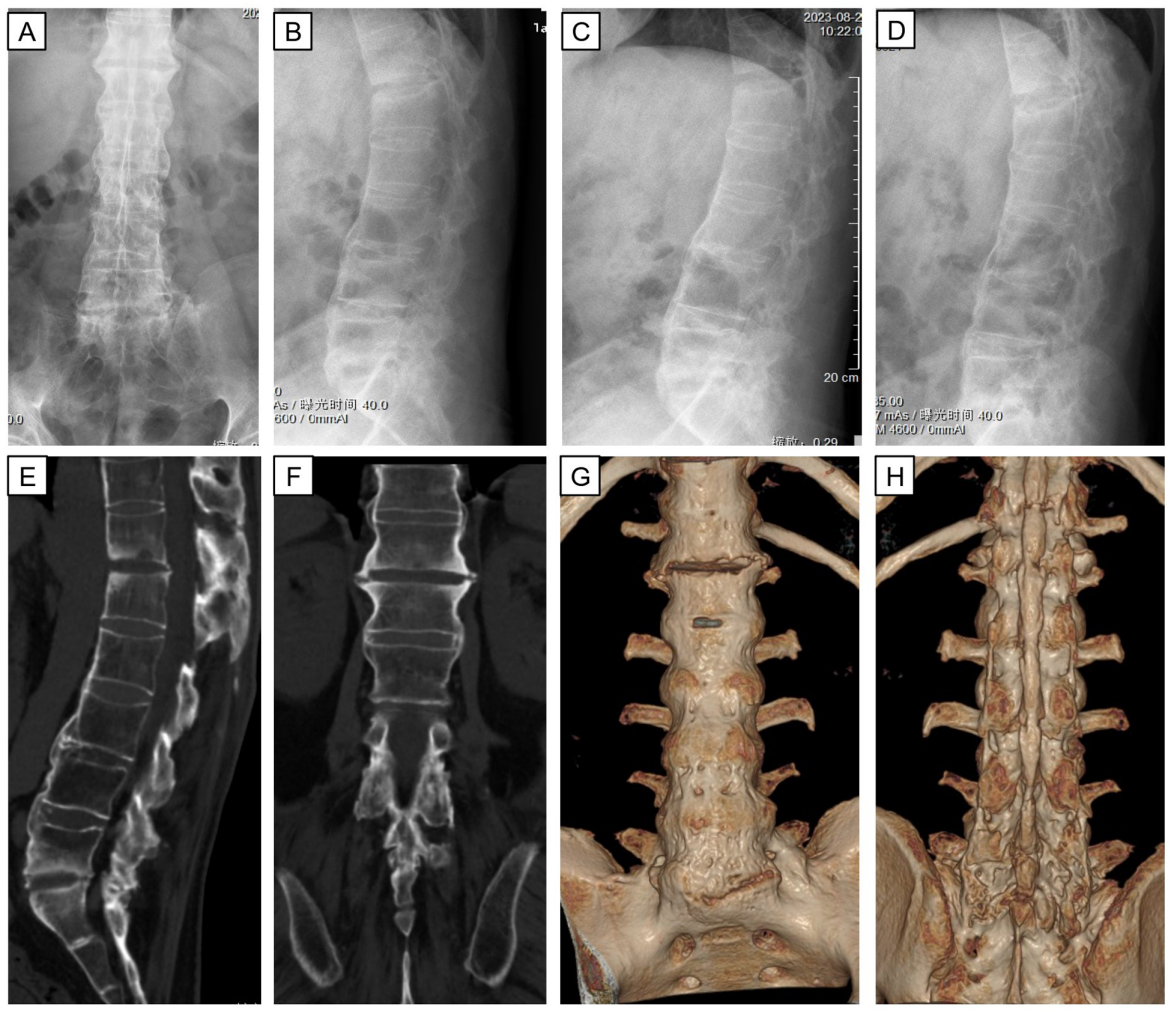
X-ray and CT three-dimensional reconstruction of thoraco-lumbar vertebrae after admission. Anterior and lateral radiographs (A,B) show abnormal paravertebral and anterior longitudinal ligament ossification. Flexion and extension lateral radiographs (C,D) represent a loss of lumbar mobility. Sagittal, coronal, and reconstructed coronal CT images (E–H) demonstrate instability of the lumbar spine result from no bone bridging between T12 and L1.
